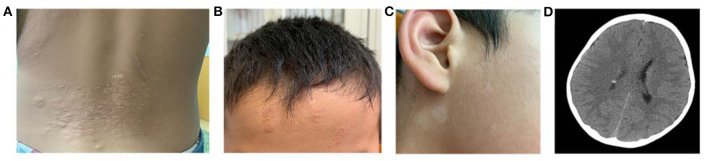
Clinical manifestations of TSC. (A) Shagreen patch at the waist of case 1. (B) Ffibrous cephalic plaque of case 2. (C) Hypomelanotic macule in the face of case 2. (D) A nodular dense shadow in the lateral wall of the right lateral ventricle in cranial CT of case 2, considered as a subependymal nodule.
